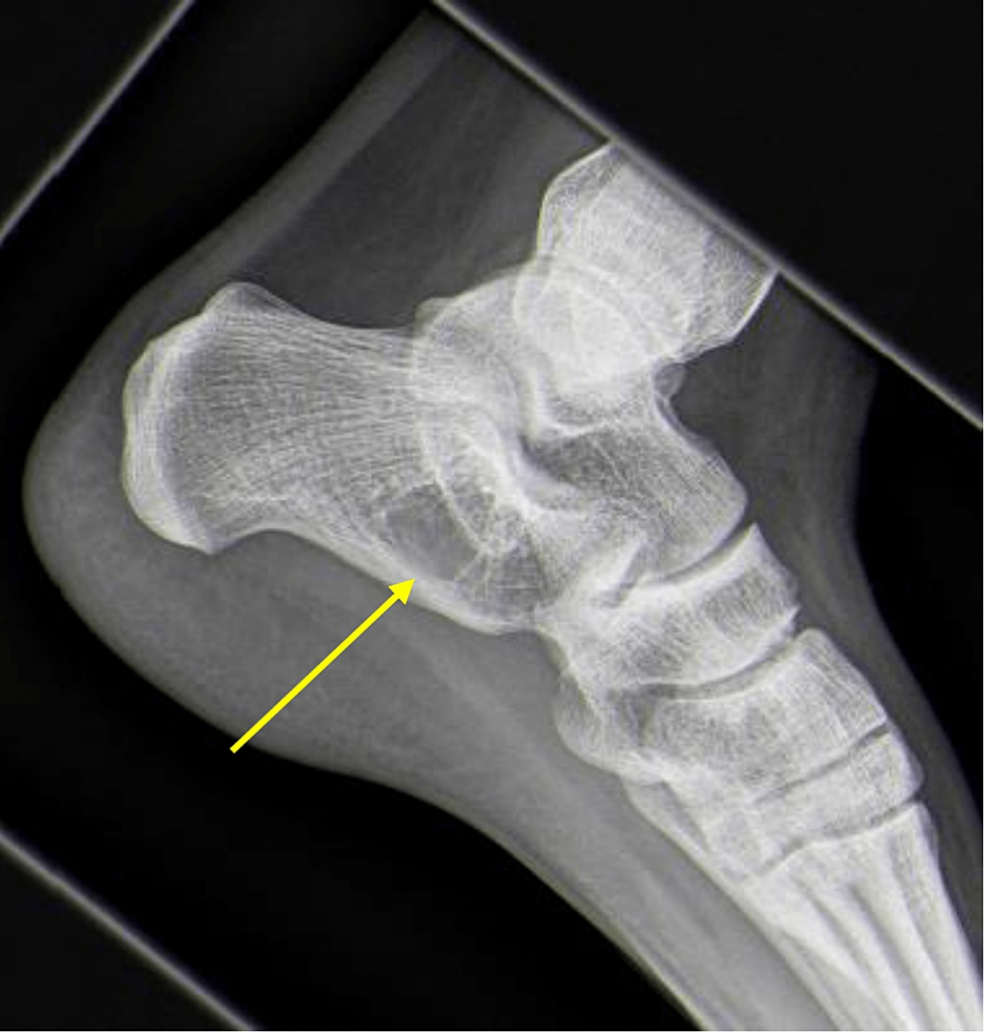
Lateral radiograph of the calcaneus from a 17-year-old patient. Radiograph demonstrates lucent lesion that was incidentally found due to metatarsal fracture from a motor vehicle collision. The lesion was determined to be a simple bone cyst after biopsy with curettage and bone grafting, which were performed due to the risk for pathological fracture.
